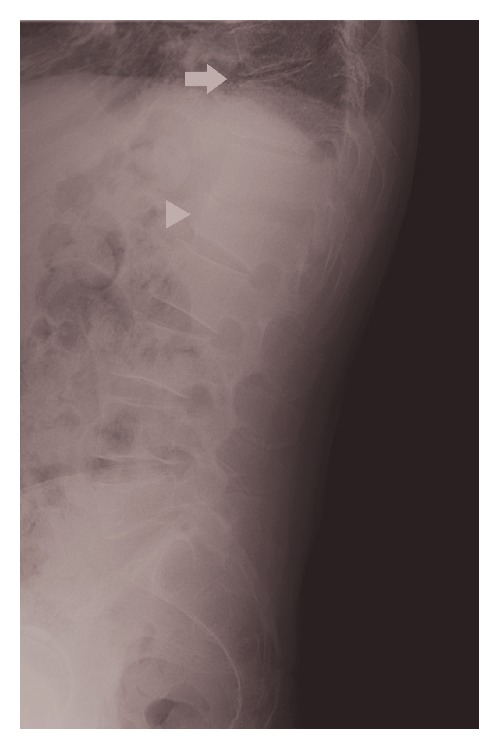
Plain radiograph of the lumbar spine revealed severe osteoarthritic changes of the thoracic and lumbar spine. There were compression collapse of T10/T11 vertebrae with mild retrolisthesis (white arrow) and a grade-one-to-two compression collapse of L1 vertebra with minimal retrolisthesis (white arrowhead).
